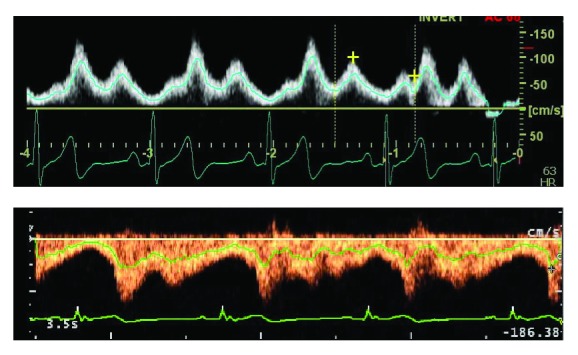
Ultrasound spectral Doppler trace of the right internal jugular vein blood flow. The mean velocity trace is highlighted.
